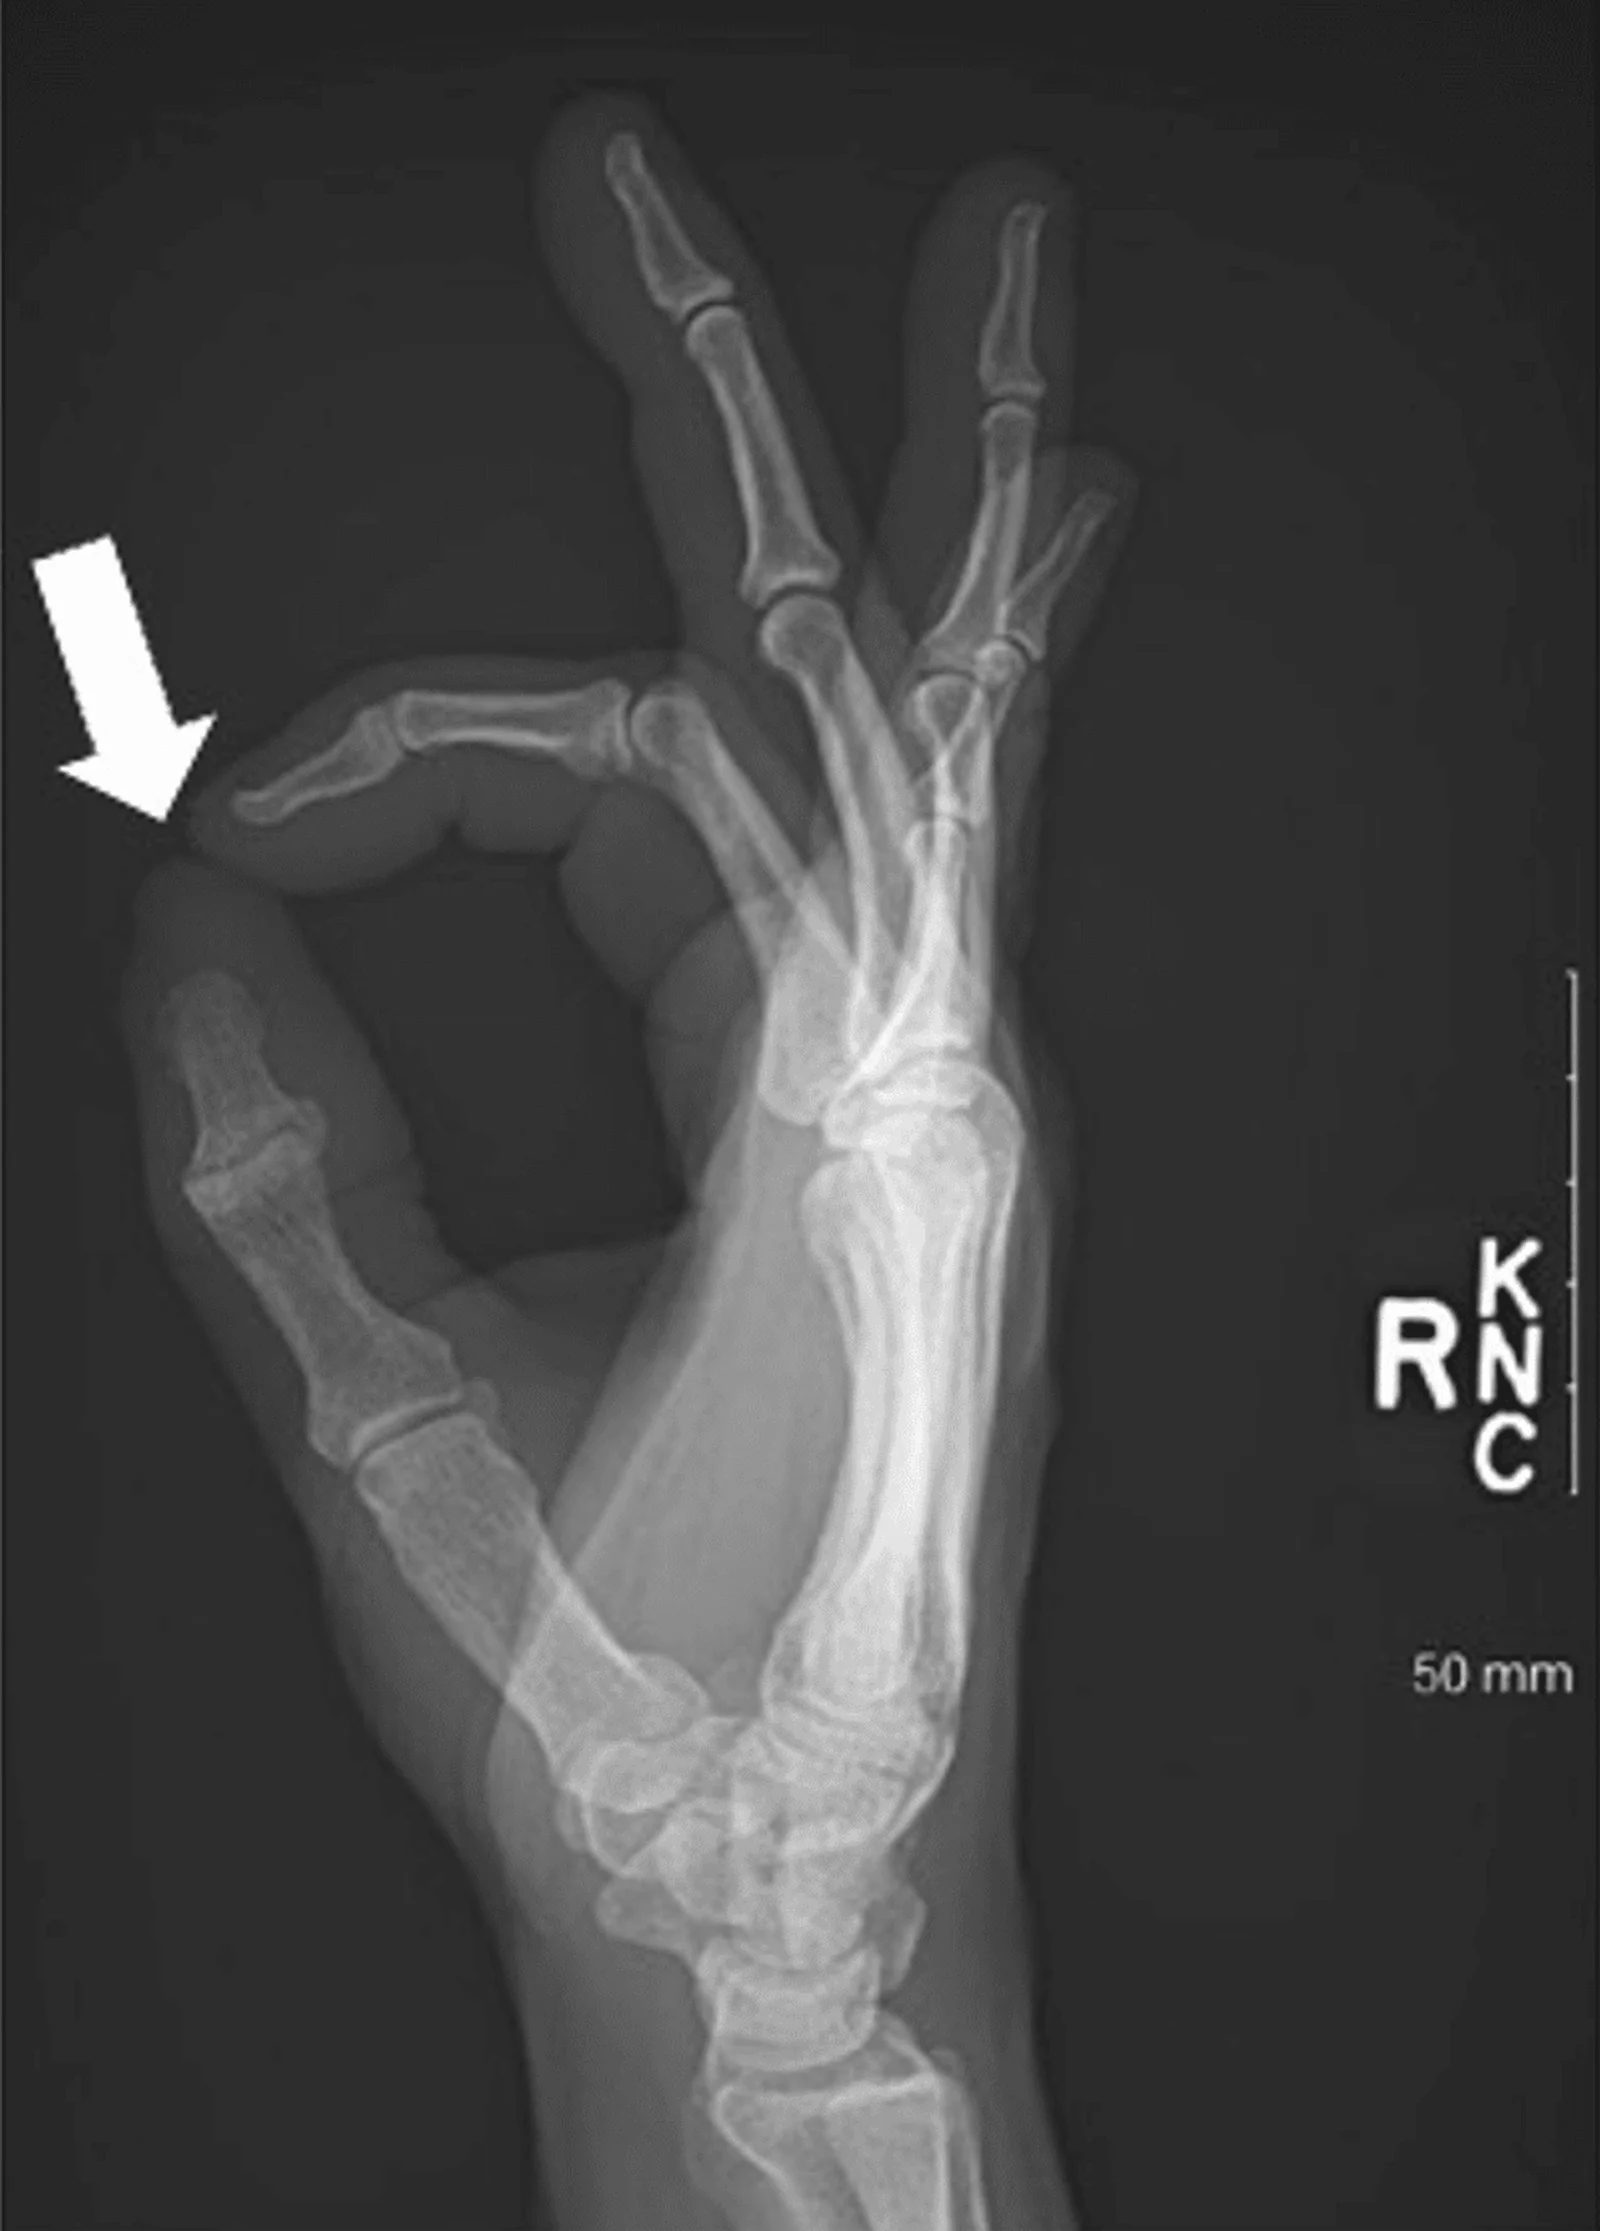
Lateral plain radiograph confirming absence of osseous erosion or periosteal reaction.
